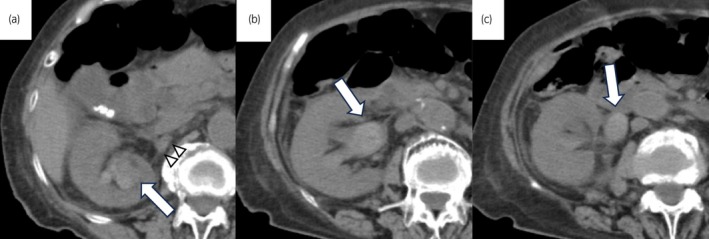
Findings of CT at acute obstructive pyelonephritis. (a) A white arrow shows a right renal pelvic tumor with active bleeding. White arrowheads show mild strandings around the renal artery. (b) A white arrow shows a dilated right renal pelvis due to blood clots. (c) A white arrow shows a dilated right ureter due to blood clots.
